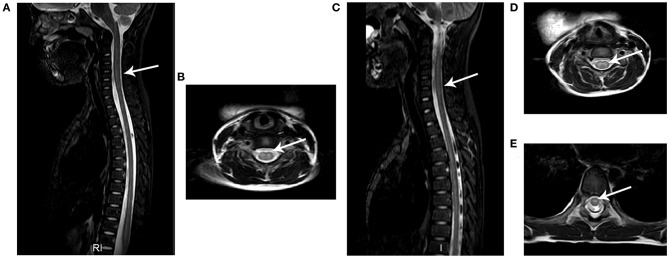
Typical MRI results of AFM patients. The images from two AFM patients (case a: A,B; case b: C,E) after onset of neurological symptoms. (A,C) Sagittal T2-weighted images showing longitudinal hyperintensity (arrow) in central gray matter. (B,D,E) Axial T2-weighted sequences showing hyperintensity in spinal cord gray matter.
